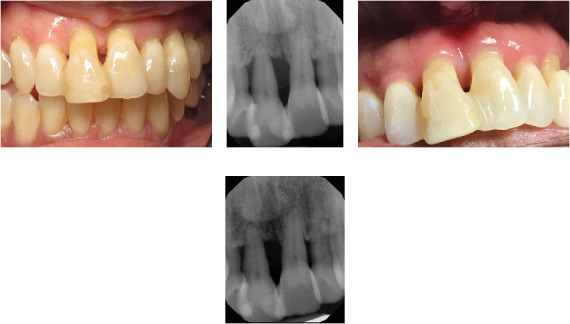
Periodontally compromised maxillary incisors were splinted in 2014 (A and B). Clinically (C) and radiographically (D) observed long-term stability in 2023.
